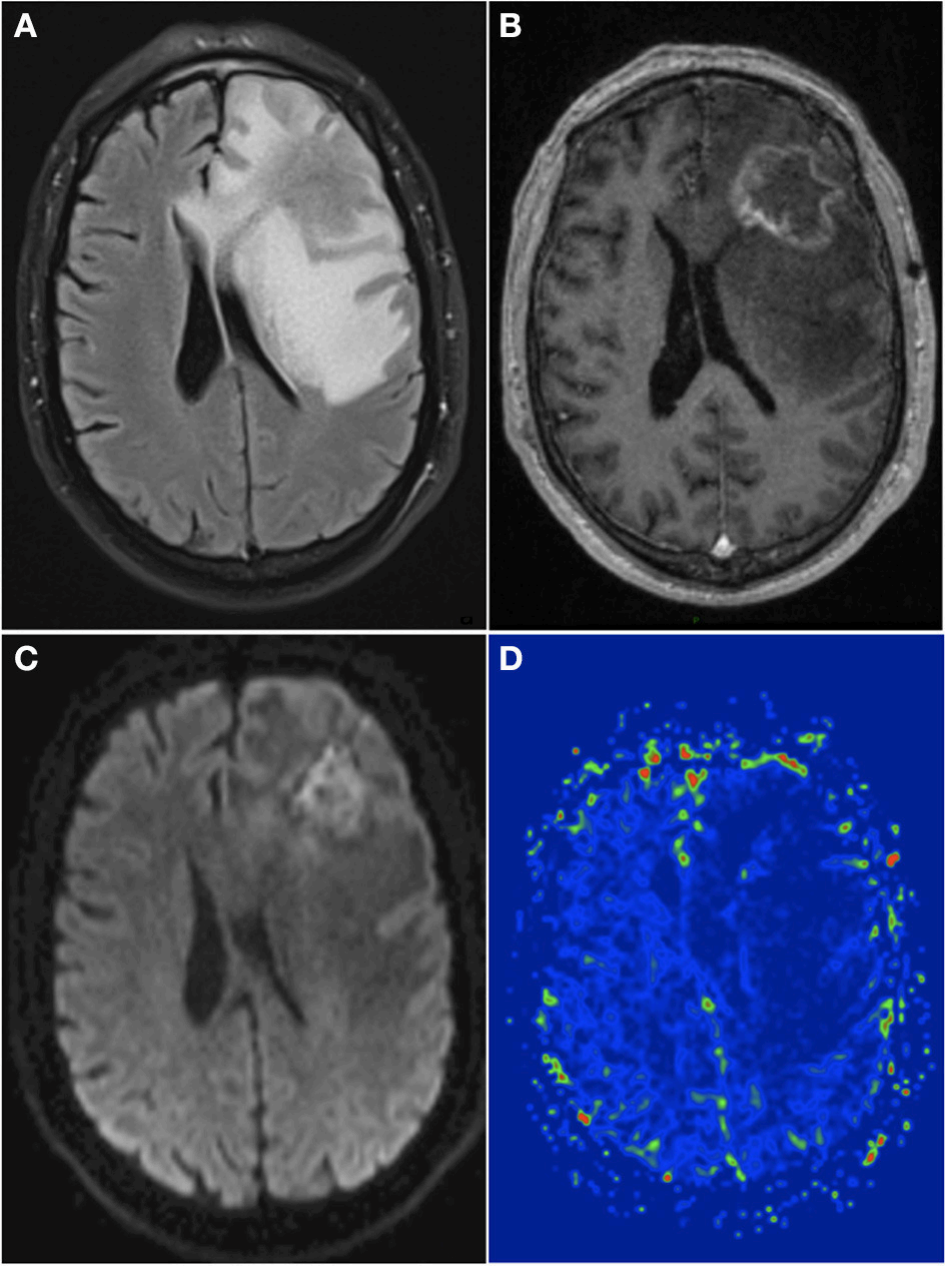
A 66-year-old man with history of brain metastasis of non-mutated NSCLC and treated by surgical resection and postop SRT. (A) Axial T2w FLAIR sequence showed a hyperintense signal appeared around the treated region 13 months after SRT. (B) T1w contrast sequence showed an inhomogeneous ring enhancement within the treated region. (C) DWI showed a low signal within the enhanced margin, with a high ADC (not shown). (D) Dynamic susceptibility contrast-enhanced perfusion weighted imaging showed a low hyperperfusion with a relative cerebral blood volume of 1.5, suggesting absence of tumor recurrence. Surgical resection confirmed the diagnosis of cerebral RN. NSCLC, non-small cell lung cancer; T2w, T2 weighted; T1w, T1 weighted; DWI, diffusion weighted imaging; ADC, apparent diffusion coefficient; SRT, stereotactic radiotherapy; RN, radionecrosis.
